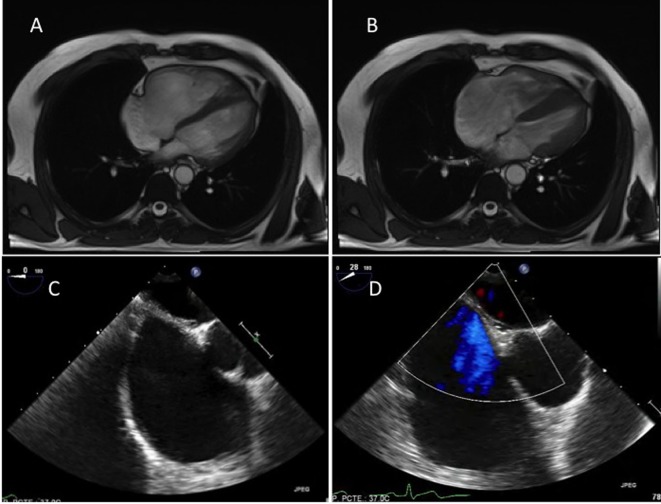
Magnetic resonance, four-chamber view: diastolic (panel A) and systolic (panel B) frame, exhibiting normal right ventricular shortening. Transesophageal echocardiography, mid-esophageal plane (panel C) and also a color-Doppler (panel D) view exhibiting no interatrial shunt.
